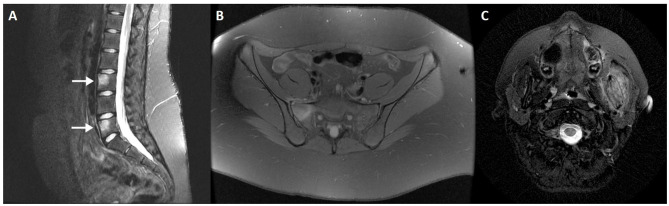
Fat-saturated T2WI sagittal MRI sequence (A) of the lumbar spine in a 16-year-old female patient with CRMO (arrows), mimicking corner inflammatory lesions. Fat-saturated PD axial slice (B) of the same patient depicting involvement of the right SIJ. Fat-saturated T2WI axial slice (C) of the neck shows involvement of the left mandibular ramus.
